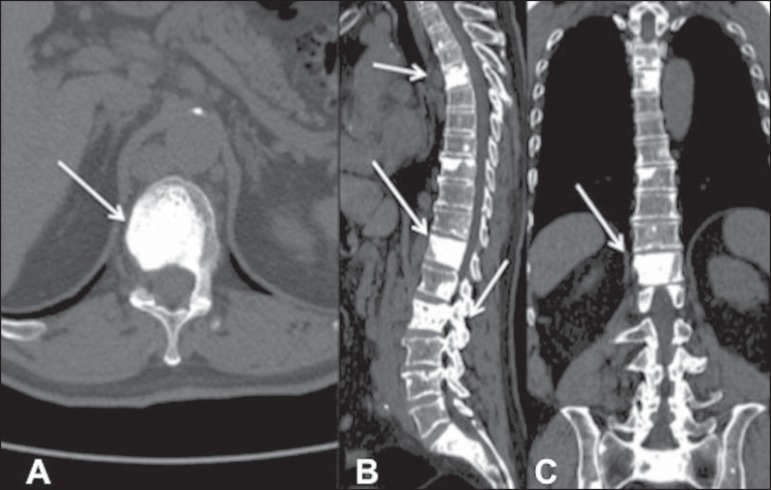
62-year-old patient with prostate cancer. computed tomography
